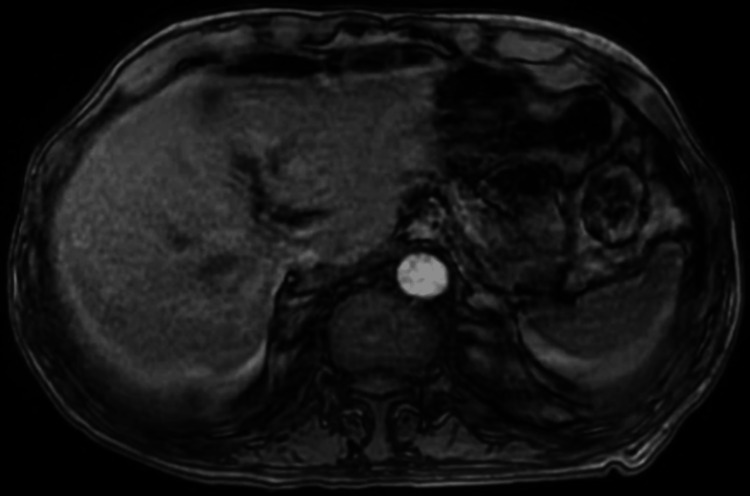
MRI of the abdomen showing no evidence of intrahepatic or common duct dilation, abnormal enhancement, mass, or extrinsic mass effect.
